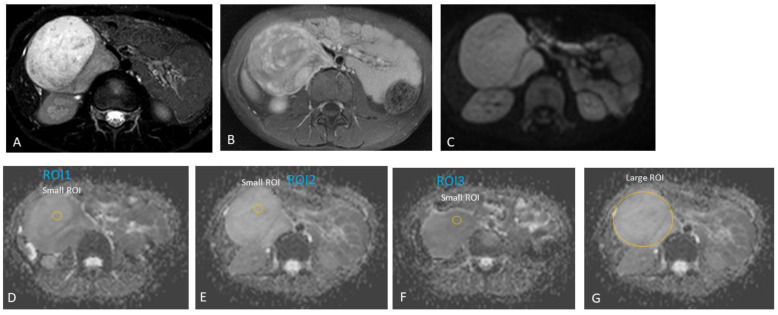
Right retroperitoneal ganglioneuroma in an eight-year-old boy. Incidental finding of an abdominal mass in the right upper quadrant on ultrasound after head injury. Heterogeneous tumour, predominantly solid. Therefore, the regions-of-interest (ROIs) were randomly placed within the tumour during the analysis of apparent diffusion coefficient (ADC) diffusion-weighted (DW) MRI values. (A) Axial T2, (B) axial post-gadolinium T1, and (C) axial DWI (b-value: 600 mm^2^/s). (D–F) Three small regions-of-interest (ROIs) on the ADC map with an area of 19.1 mm^2^; ADC for the small ROI method, 1.75 × 10^−3^ mm^2^/s. (G) One large ROI on the ADC map with an average area of 2990.7 mm^2^; ADC for the large ROI method, 2.55 × 10^−3^ mm^2^/s.
